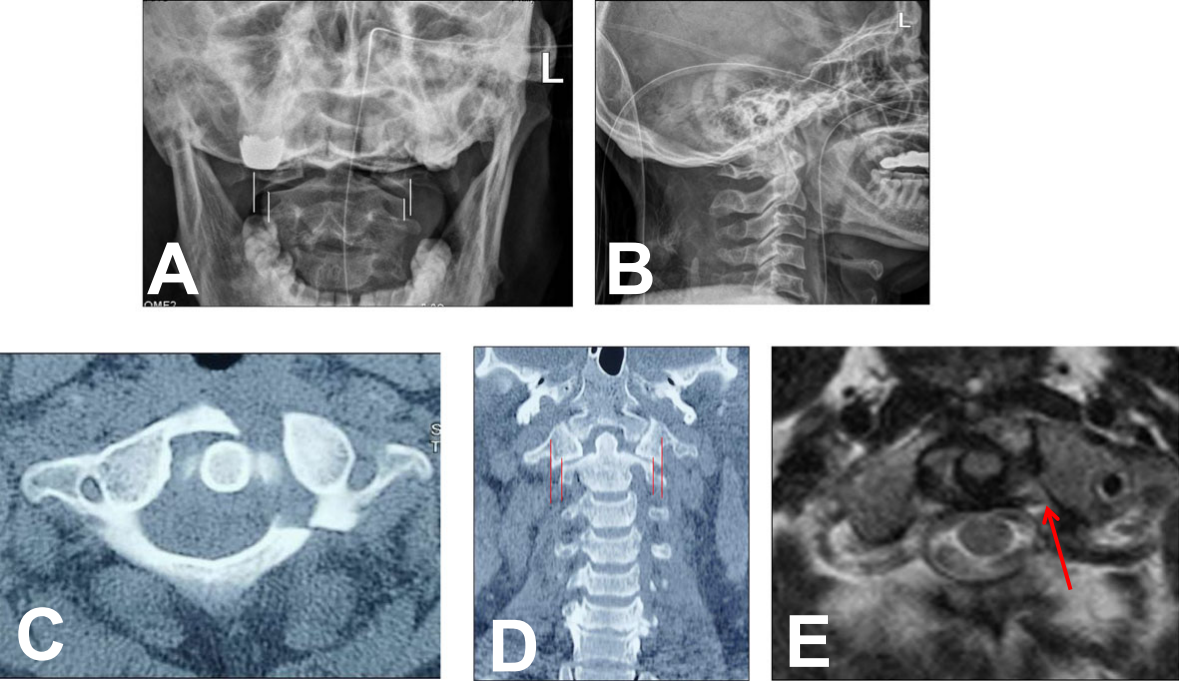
A represent case (A, B, C, and D) Preoperative X-ray and CT showed C1 fracture. C There were fracture lines in both anterior and posterior arches (Gehweiler type III). The LMD value measured on the open X-ray film was 7.28 mm (A), and the value measured on the coronal CT film was 6.54 mm (D). The MRI showed that the TAL had a high signal (red arrow) at the attachment point of the left lateral mass, considering an avulsion injury (Dickman type II). While compression of the spinal cord was not observed (E)
